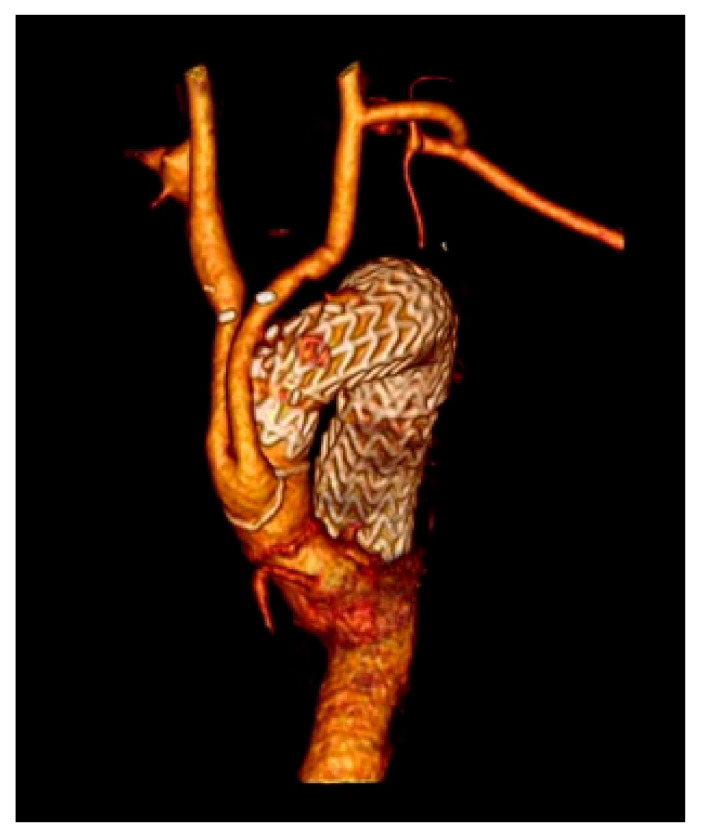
3 years follow-up type II debranching CT scan.
